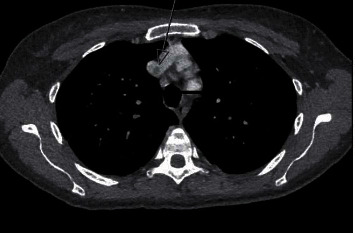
Axial computed tomography angiography depicting a filling defect (arrow) in the upper superior vena cava at the junction of the left and right brachiocephalic veins.
